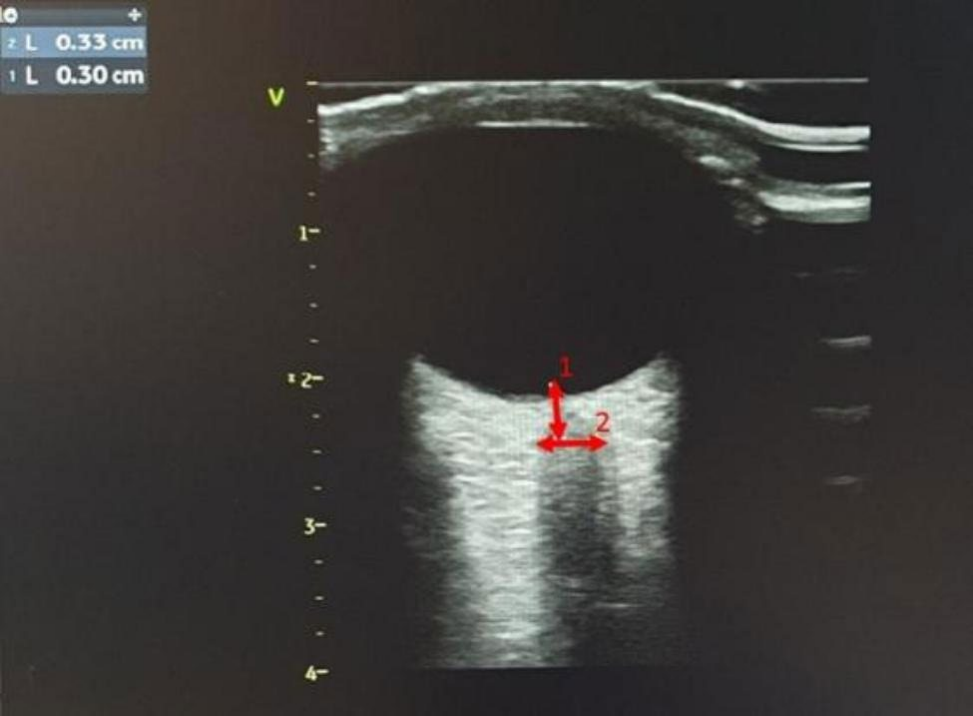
Ultrasonographic image of optic nerve sheath diameter measurement. (1. Distance behind the optic disc where the optic nerve sheath diameter (ONSD) is measured in its width, 2. ONSD measurement)
